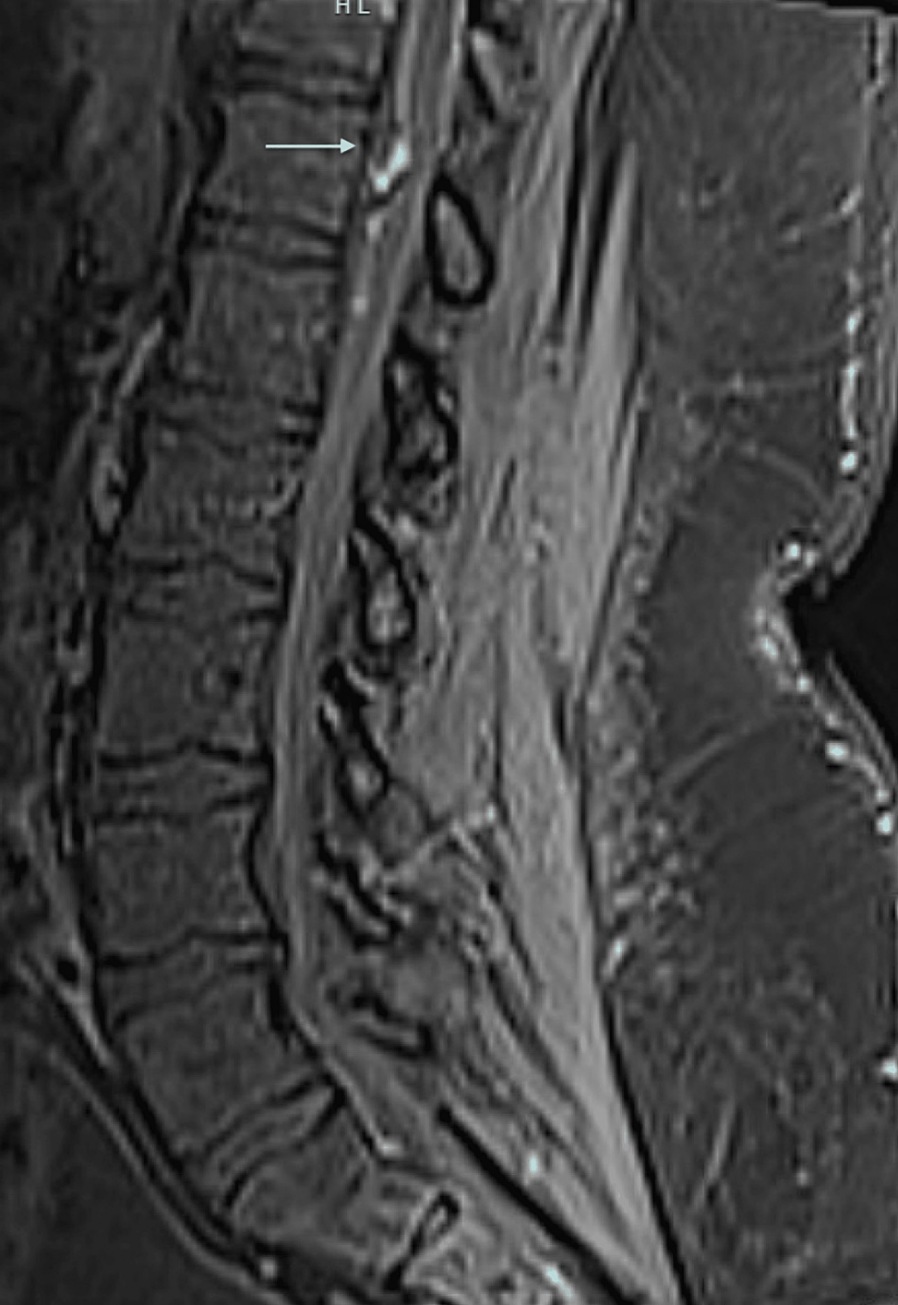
Preoperative Magnetic Resonance Imaging of the Spinal Cord Non-homogeneous intramedullary extramedullary lesion measuring 1.3 cm at T12.
